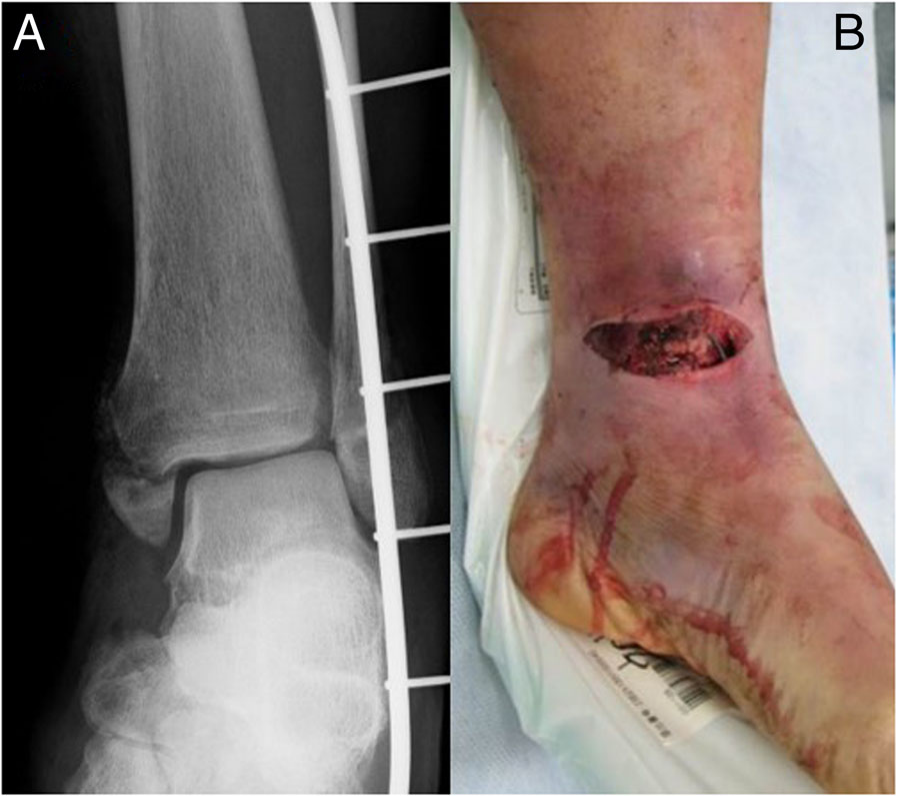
Anteroposterior plain radiography of the *left ankle* (a) and physical appearance of the open wound (b). The injury was classified as a Gustilo–Anderson 3B, AO classification 44-B2 open fracture
